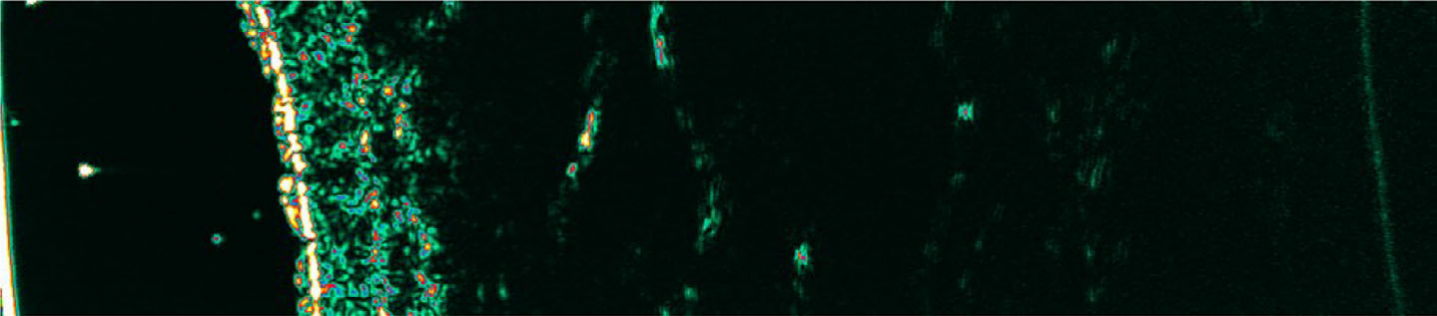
High‐frequency ultrasound image in a patient with acute cutaneous lupus erythematosus (cheek skin). All layers of the skin are visible and the echogenicity of the skin is reduced.
